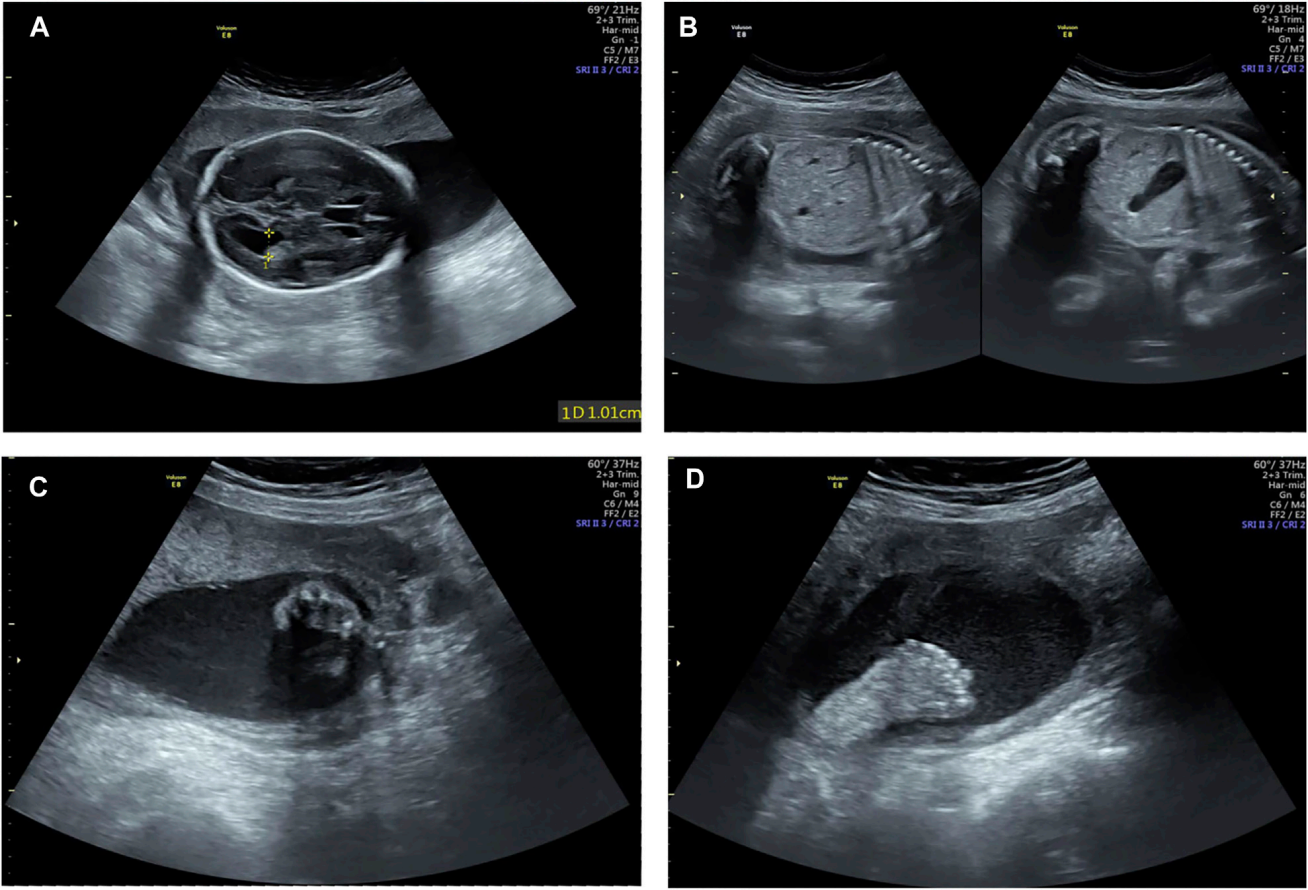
Examination image of the fetus. Fetal ultrasound scan showed fetus malformation including lateral ventricle widening (A), bilateral renal echo enhancement (B), and polydactyly (C,D).
